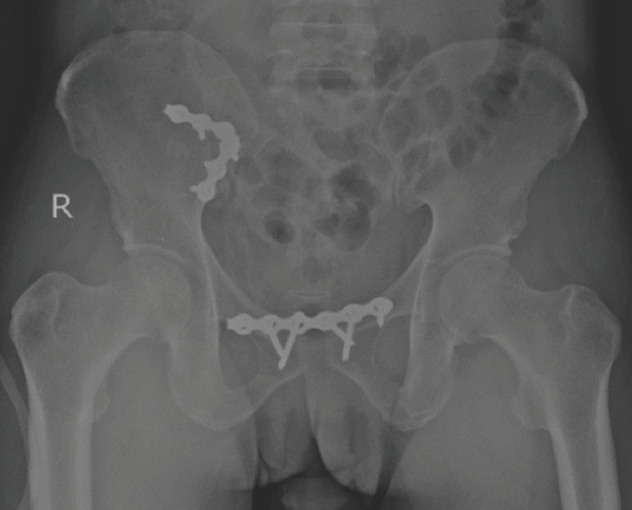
Post-operative pelvic radiograph.
